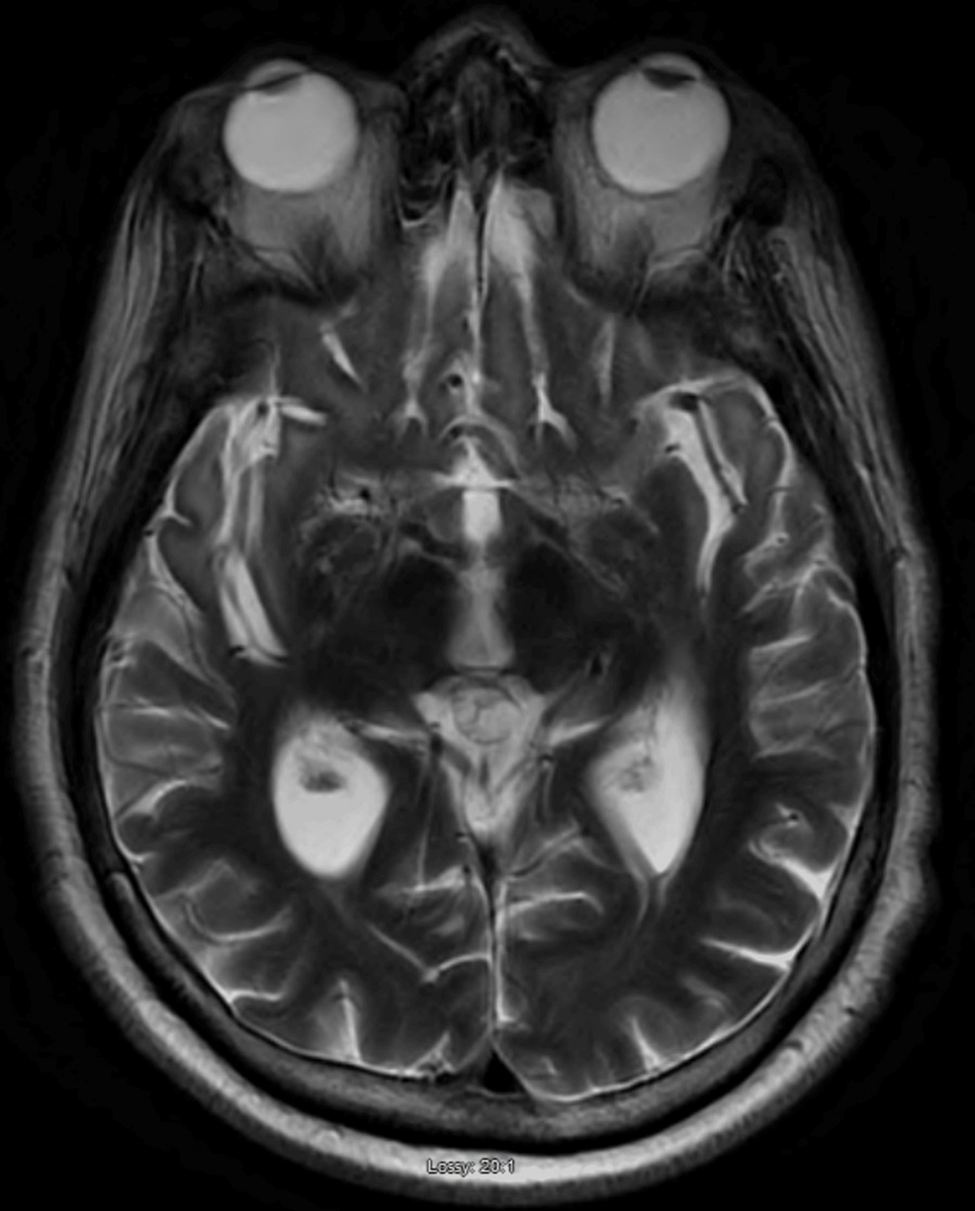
MRI with diffuse cerebral volume loss
